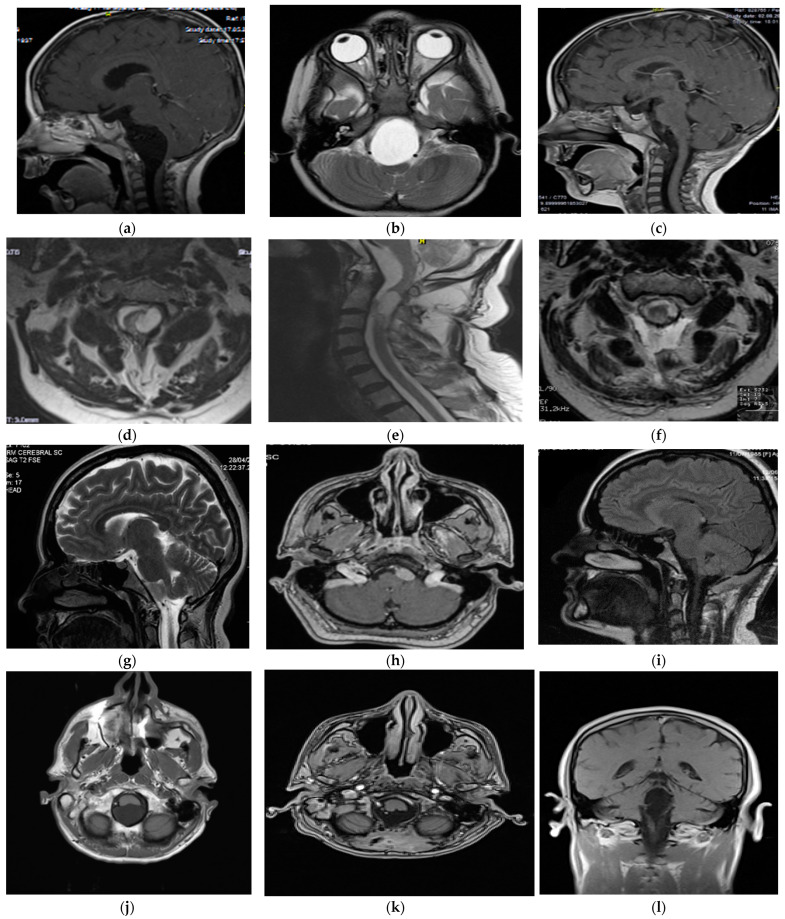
Preoperative and postoperative MRI characteristics of personal case series. Patient #2 (CVJ neurenteric cyst)—preoperative MRI (image (a)—saggital cut, T1-weighted; image (b)—axial cut, T2-weighted) showing a hypointense mass in the pontomedullary cistern extending to C1; 3-month follow-up MRI (image (c)) demonstrating the extent of the gross total resection. Patient #3 (CVJ dermoid cyst)—preoperative MRI (image (d)—axial cut, T2-weighted; image (e)—saggital cut, T2-weighted) showing a hyperintense mass in the premedullary cistern extending to C2; 3-month follow-up MRI (image (f)—axial cut, T2-weighted) demonstrating extent of subtotal resection. Patient #4 (CVJ neuroenteric cyst)—preoperative MRI (image (g)—saggital cut, T2-weighted; image (h)—axial cut, T1-contrast) showing a hypointense mass without enhancement in the premedullary region; 3-month follow-up MRI (image (i)—saggital cut, T1-weighted) demonstrating the extent of gross total resection. Patient #6 (CVJ epidermoid cyst) — preoperative MRI (image (j)—axial cut, T2-weighted) revealed a hyperintensity mass in the cerebellomedullary cistern extending to C1; 3-month follow-up MRI (image (k)—axial cut, T1 contrast, image (l)—coronal cut, T1-weighted) demonstrate gross-total resection.
